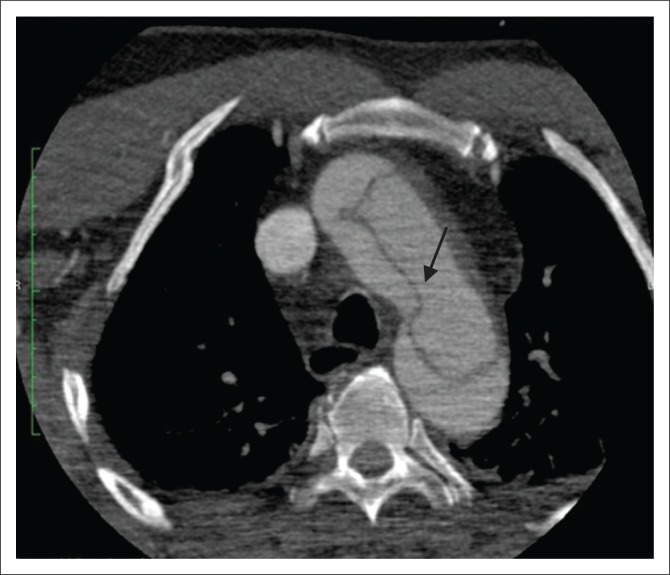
Contrast-enhanced axial computed tomography scan demonstrating a displaced intimal flap (arrow), separating the true and false lumens in a patient with a Stanford Type A aortic dissection.
